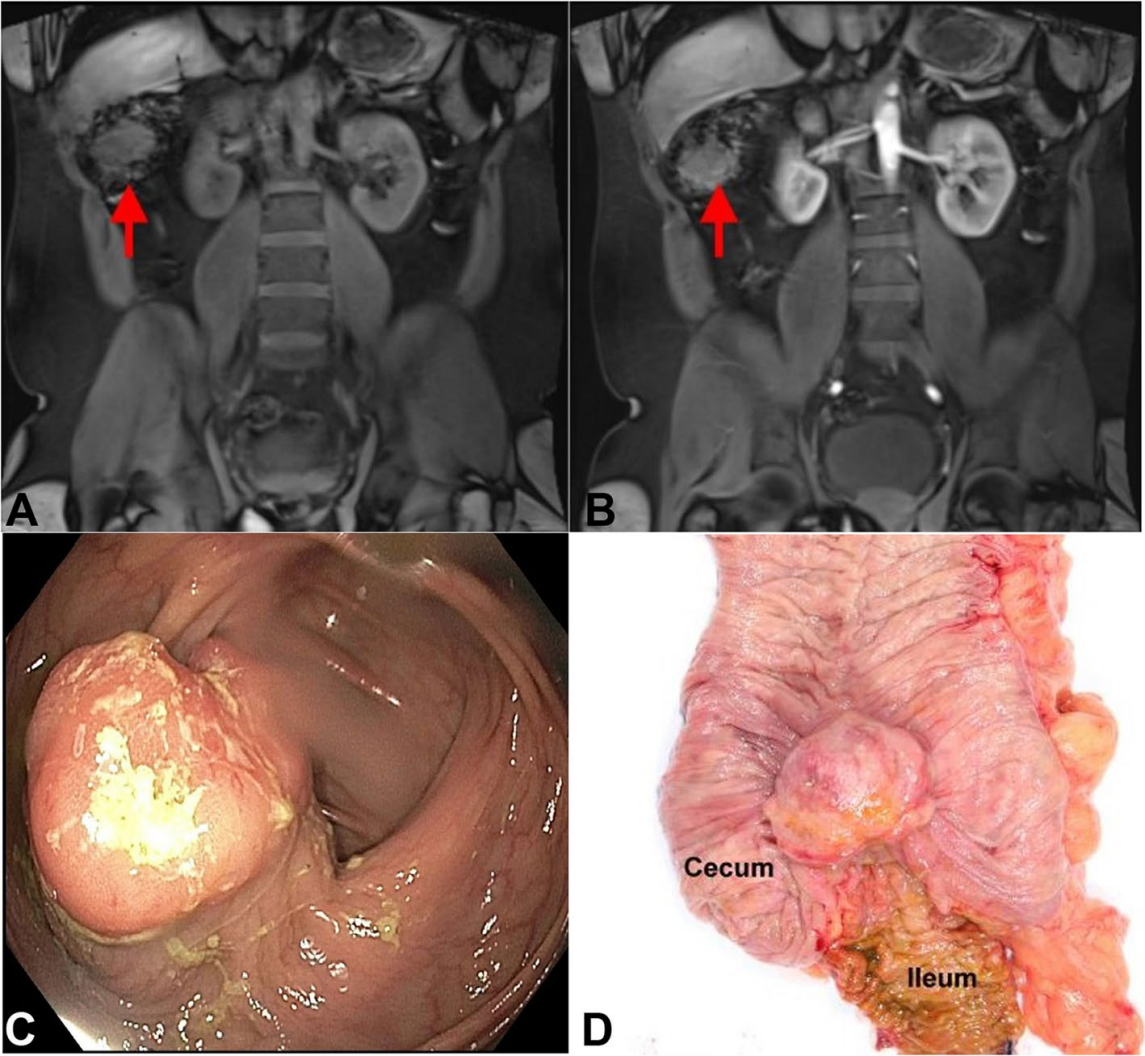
A Unenhanced MRI showed relative low T1W signal (arrow) in the tumor. B. Arterial phase showed early heterogeneous enhancement (arrow). C The tumor was masqueraded under colonoscopy as edematous and inflamed ileocecal valve with overlying frond-like/villous mucosa. D Right colectomy showed a tan ulcerated polypoid mass close to ileocecal valve protruding into the mucosal surface
